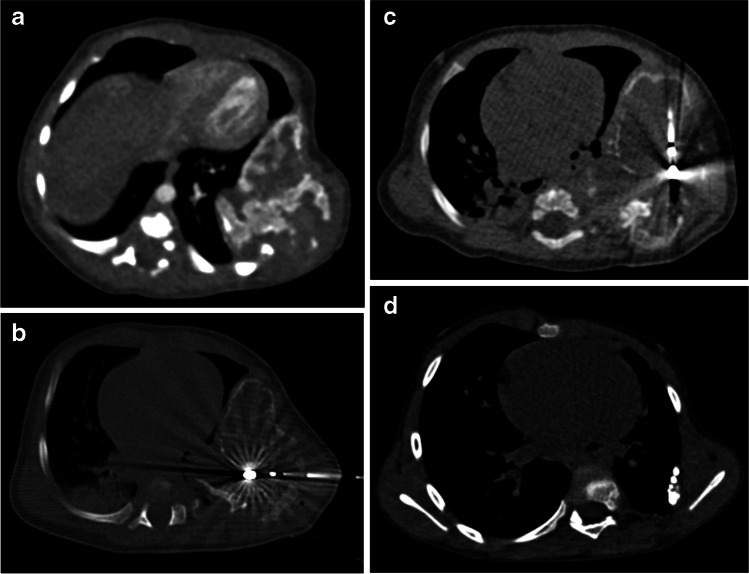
Mesenchymal hamartoma in a 2-week-old boy at diagnosis (patient 1) treated with microwave thermoablation. a Axial CT scan with contrast agent shows an inhomogeneously hypodense oval lesion involving the 5th–6th left ribs. b, c The boy underwent two microwave thermoablation sessions (axial CT images) followed by a dimensional increase in the mass, requiring surgical resection. d After 21-month follow-up, axial contrast-enhanced CT scan demonstrates almost complete resection of the lesion
